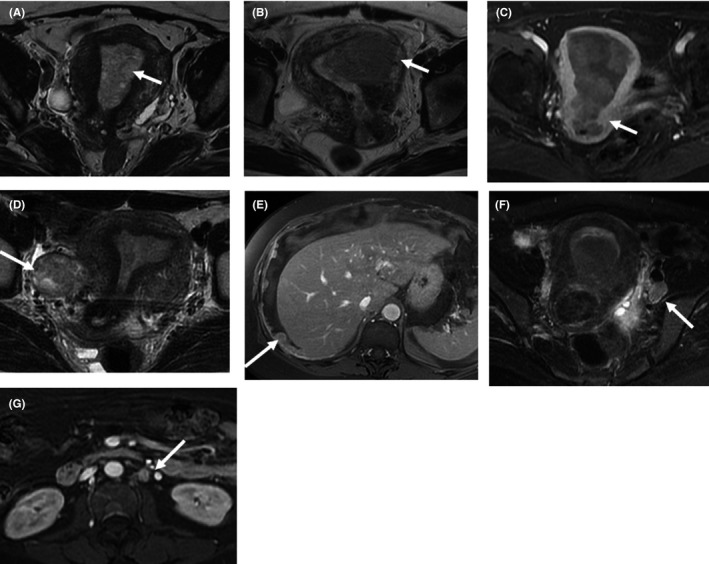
Magnetic resonance imaging of different pathological parameters. (A) Coronal oblique T2‐weighted image of the uterus showing endometrial cancer with superficial myometrial invasion in a 47‐year‐old woman. The endometrial tumor exhibits <50% myometrial invasion (arrow). (B) Coronal oblique T2‐weighted image of the uterus showing endometrial cancer with deep myometrial invasion in a 60‐year‐old‐woman. The endometrial tumor exhibits ≥50% myometrial invasion (arrow). (C) Axial post‐contrast T1‐weighted image of endometrial cancer with cervical invasion (arrow) in a 69‐year‐old woman. (D) Coronal oblique T2‐weighted image of the uterus showing endometrial cancer with adnexal metastasis in a 33‐year‐old woman. The endometrial tumor (arrow) is shown with a solid right adnexal tumor (arrowhead). (E) Axial post‐contrast T1‐weighted image of endometrial cancer with intra‐abdominal metastases in a 50‐year‐old woman with moderate ascites. Note: The multiple peritoneal tumors at the perihepatic region (arrows). (F) Axial T2‐weighted image with fat saturation of endometrial cancer with pelvic lymph node metastases in a 37‐year‐old woman. Note: The enlarged lymph node at the left external iliac region (arrow). (G) Axial post‐contrast T1‐weighted image of endometrial cancer with para‐aortic lymph node metastases in a 63‐year‐old woman. Note: The enlarged lymph node at the para‐aortic region (arrow)
